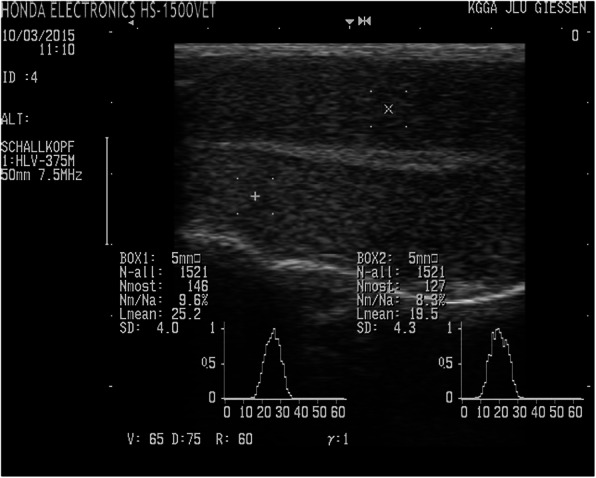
Longitudinal ultrasonography of a testis from a buck of the altrenogest group (HS 1500 V, Honda Electronics). Two regions of interest were marked to perform a grey-scale analysis of the parenchyma. 1: tunica vaginalis, 2: testicular parenchyma, 3: mediastinum
